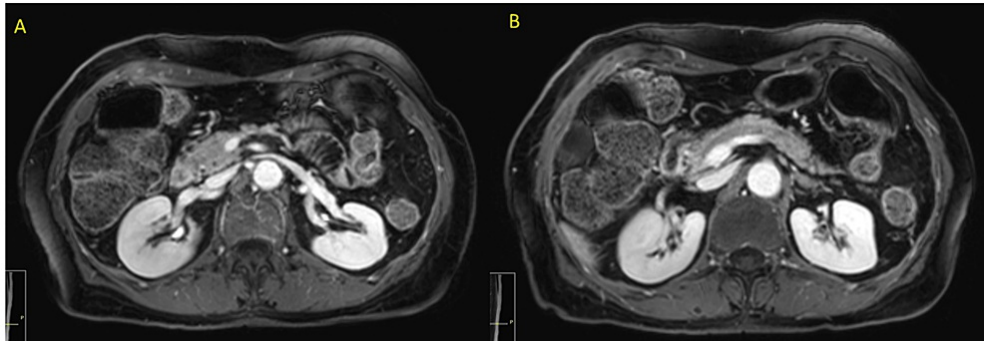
Restaging MRI Restaging MRI of the primary tumor site (A) and the prior site of the metastatic lymph node (B) demonstrating the best response to treatment. Radiographic CR was achieved with regression of the primary tumor and disappearance of the concerning lymph node. CR was later confirmed by surgical pathological evaluation. MRI: magnetic resonance imaging, CR: complete response
